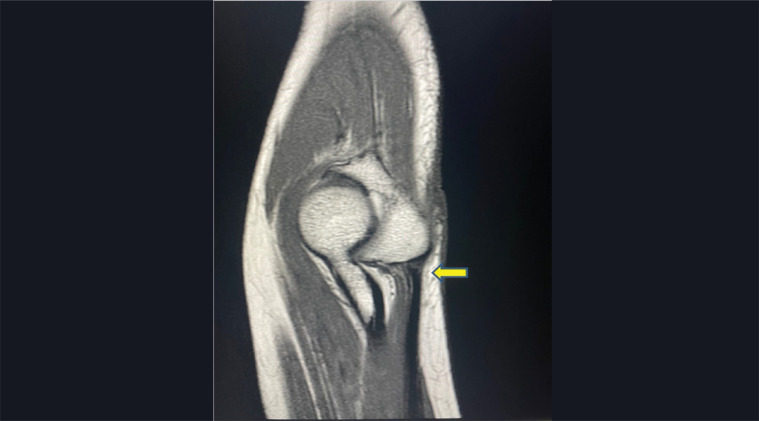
T1 Sequence MRI sagittal scan showing of the distal triceps insertion in the olecranon (yellow arrow).
